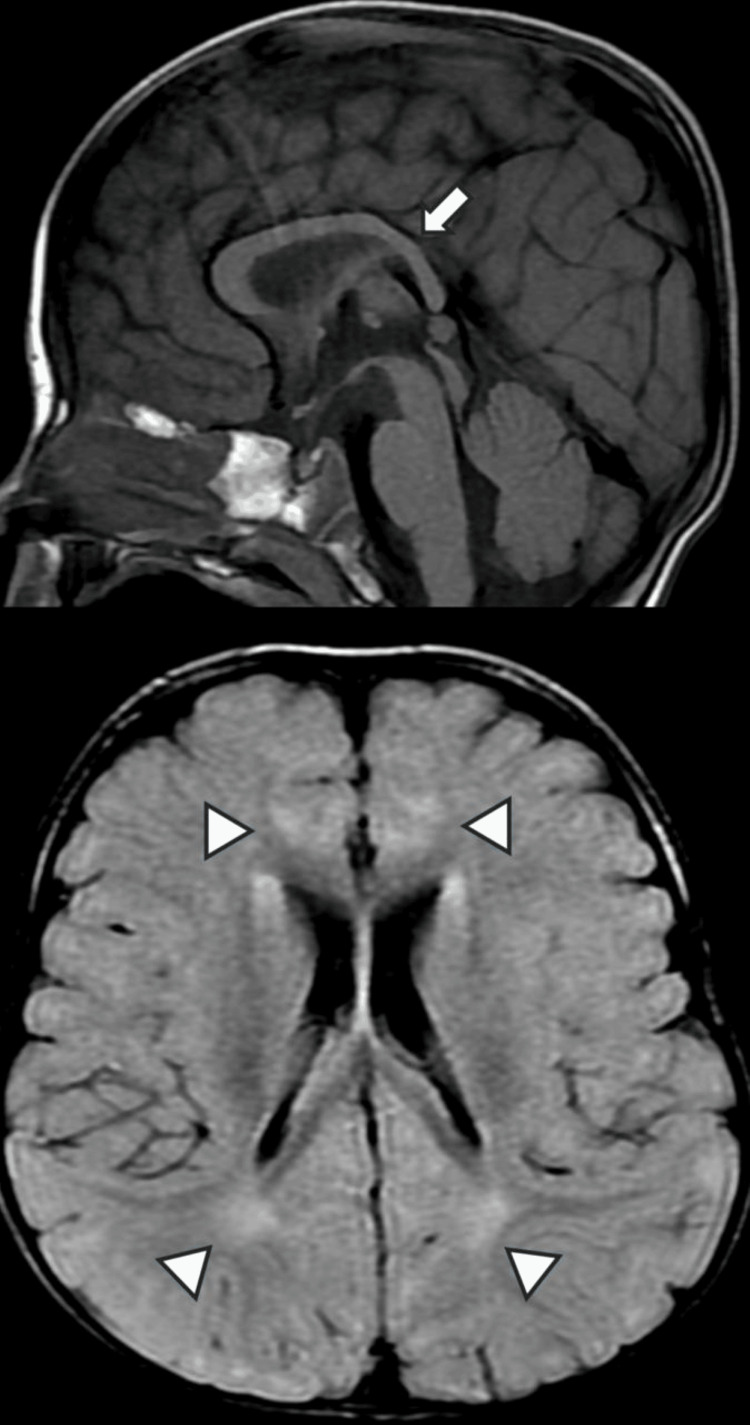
Brain MRI of case 2: Upper image of the midline sagittal T1 shows a thin corpus callosum, particularly the posterior body and splenium (white arrow). The lower axial flair image shows the reduced volume of white matter with abnormal hyperintensity of periventricular and deep white matter (white arrowheads)
